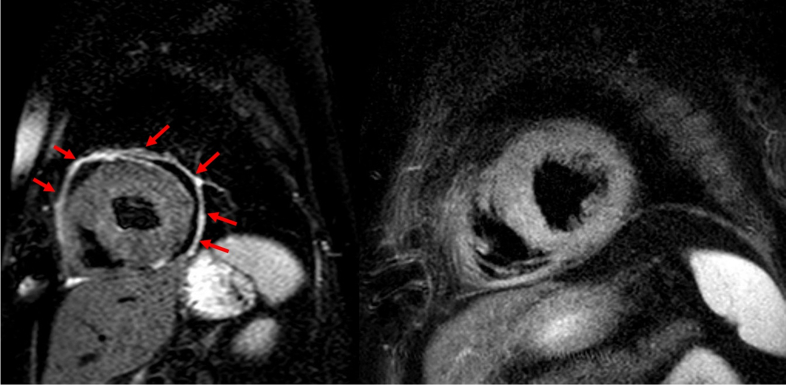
Case Presentation #3 A 53-year-old female with a history of hypertrophic cardiomyopathy who developed recurrent pericarditis following pericardiotomy (septal myectomy). The image on the left is a short-axis slice of cardiac magnetic resonance imaging showing late gadolinium enhancement suggestive of inflammation (arrows). The image on the right is repeat imaging in the same patient 1 year later with improved enhancement after completion of treatment with NSAIDs, colchicine, and prednisone. Abbreviation as in Figure 3.
